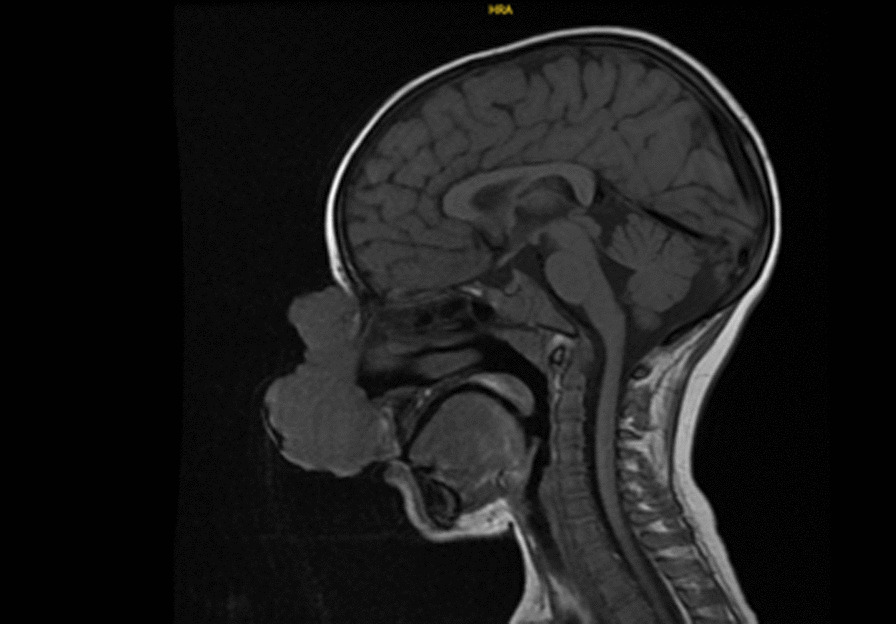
T1 sagittal magnetic resonance imaging of the brain; a lobulated giant mass that causes narrowing of the right nasal aperture with external pressure, located on the skin, starting from the level of the nasal root and extending to the level of the right nasal aperture
